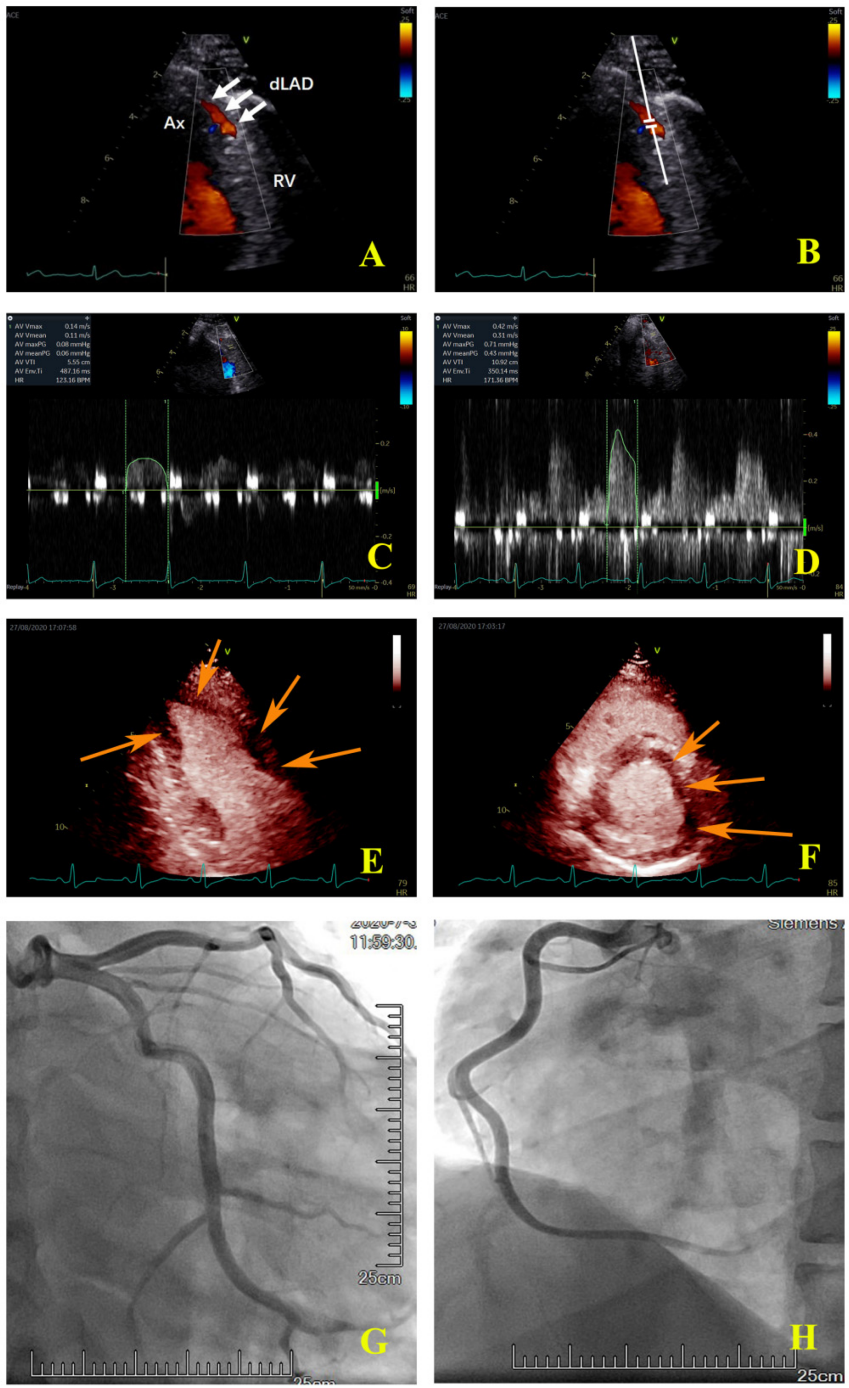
Coronary colour Doppler and blood flow spectrum and MCE and angiographic images of the same patient. (A) The colour Doppler in the distal left anterior descending artery (white arrow); (B) the sample volume was placed in the distal left anterior descending artery; (C) the coronary flow velocity in the distal left anterior descending artery at rest; (D) the coronary flow velocity in the distal left anterior descending artery at peak after stress, CFVR = 2.81; (E) the apical two-chamber view showed that the myocardial perfusion of LV apical and anterior wall was sparse (orange arrow); (F) the middle section of the LV short axis view showed that the myocardial perfusion of the anterior wall and anterior septum was sparse (orange arrow); (G) the left coronary artery angiography. (H) the right coronary artery angiography. Ax, apex; RV, right ventricle; dLAD, the distal left anterior descending artery.
